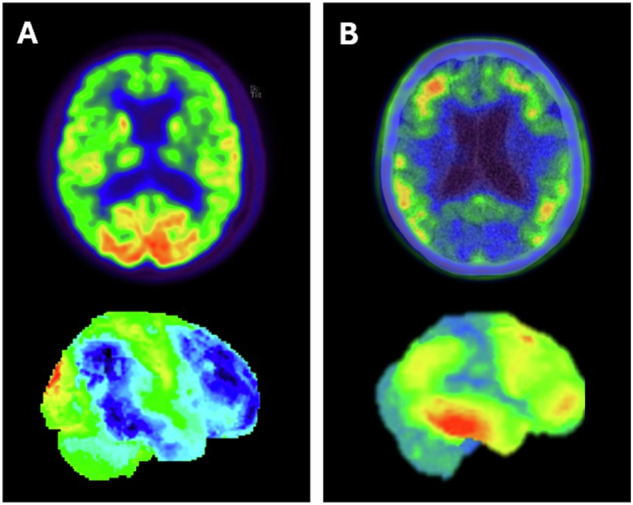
[^18^F]FDG PET (A) and [^18^F]flortaucipir (B) in an AD patient. Images show temporoparietal and frontal hypometabolism (A), which correlates with AD-related tau deposits
